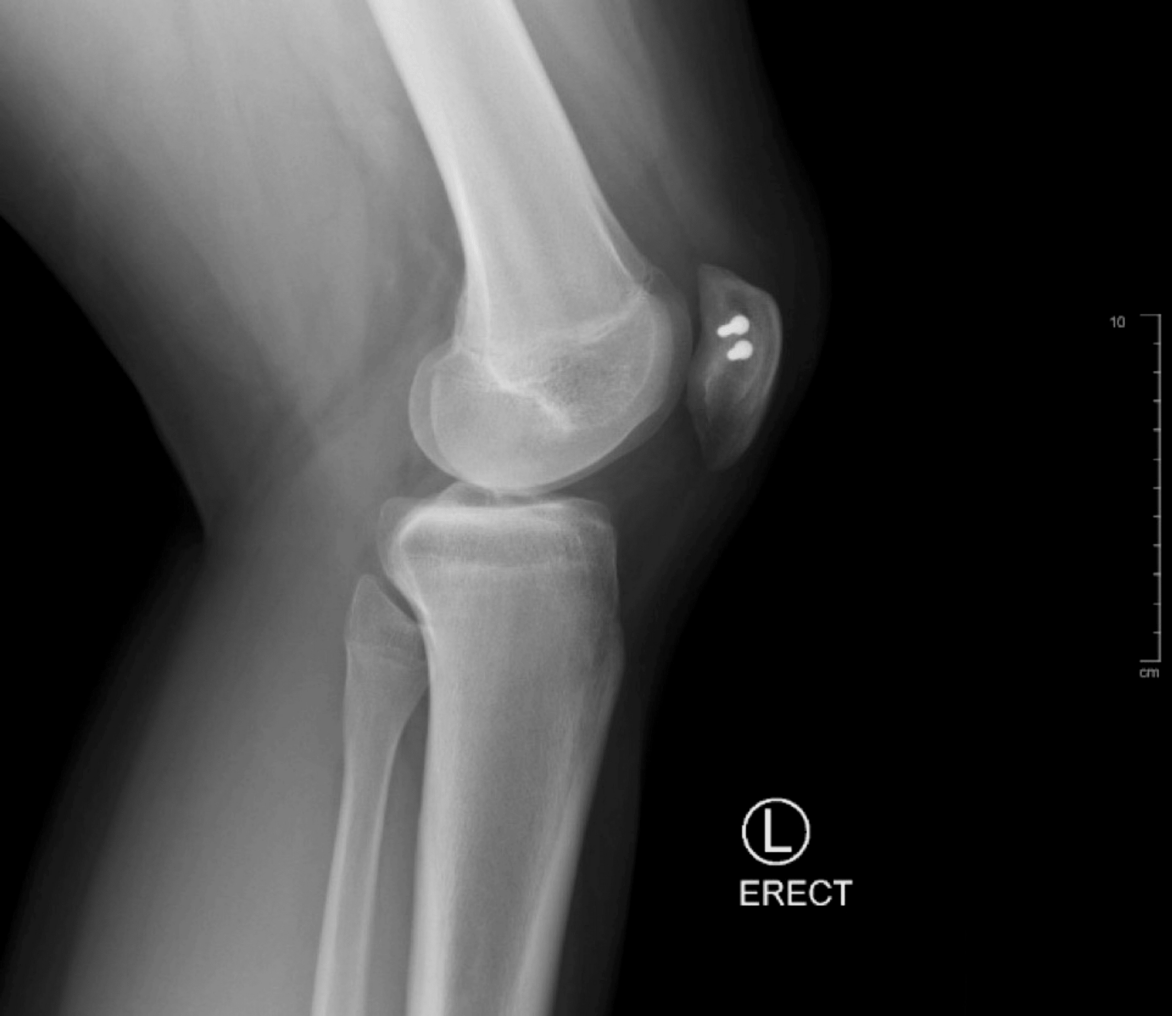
Six-month postoperative X-ray L knee erect view
